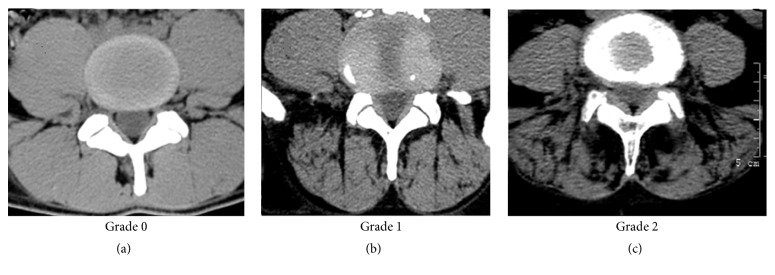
An example of different fatty infiltration grades in lumbar paraspinal muscles observed on a lumbar spine CT, imaged with a 64-slice CT scanner (Philips Medical, Brilliance Power 64). (a) A 23-year-old male; (b) a 61-year-old male; (c) a 72-year-old female.
